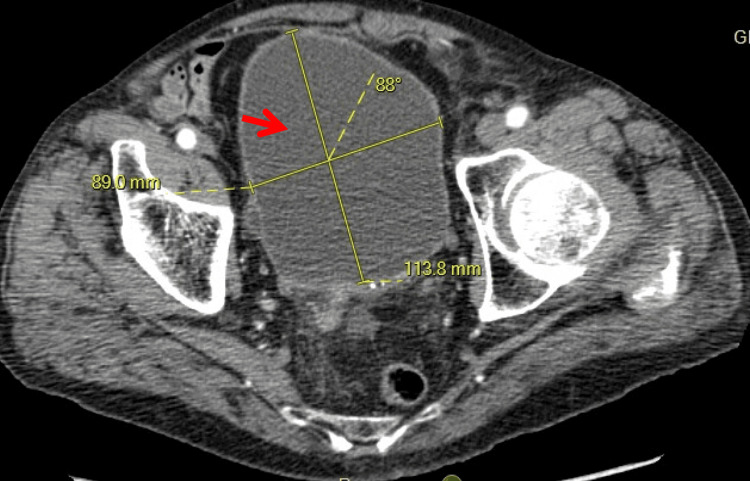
CT abdomen and pelvis, axial view. Markedly distended urinary bladder (arrow), consistent with acute urinary retention
